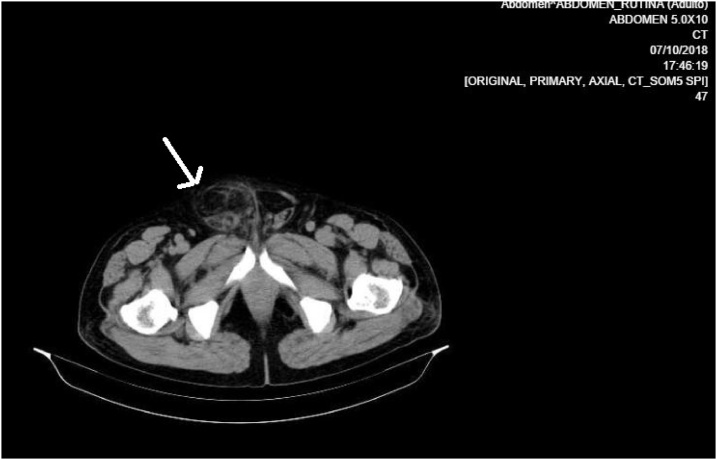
The CT scan showed the Amyand’s hernia.
